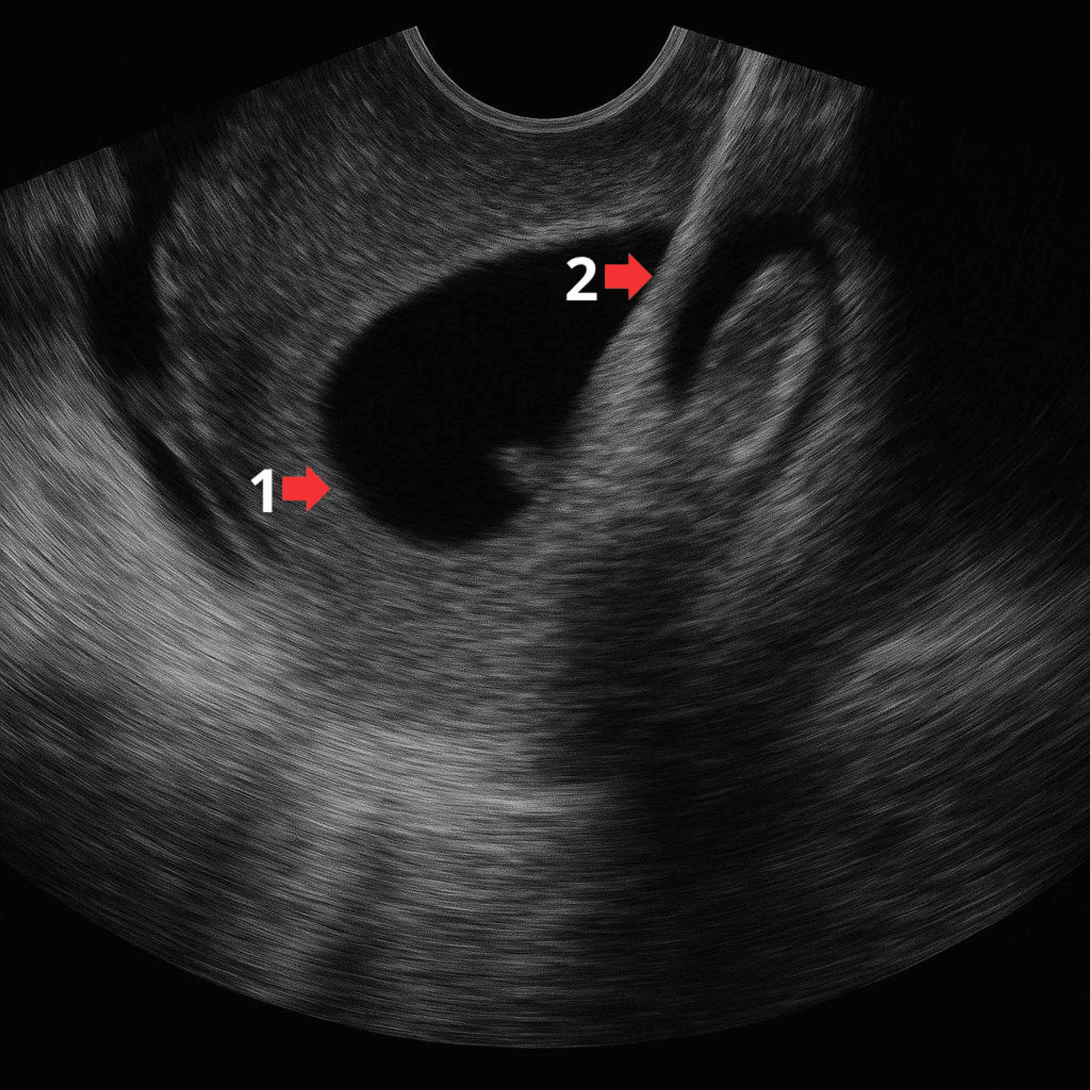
Tuning, deployment, and positioning of the distal cup or bell of the Hot Axios prosthesis in a pancreatic collection (red arrow 1); Pancreatic collection seen by endosonography (red arrow 2)
